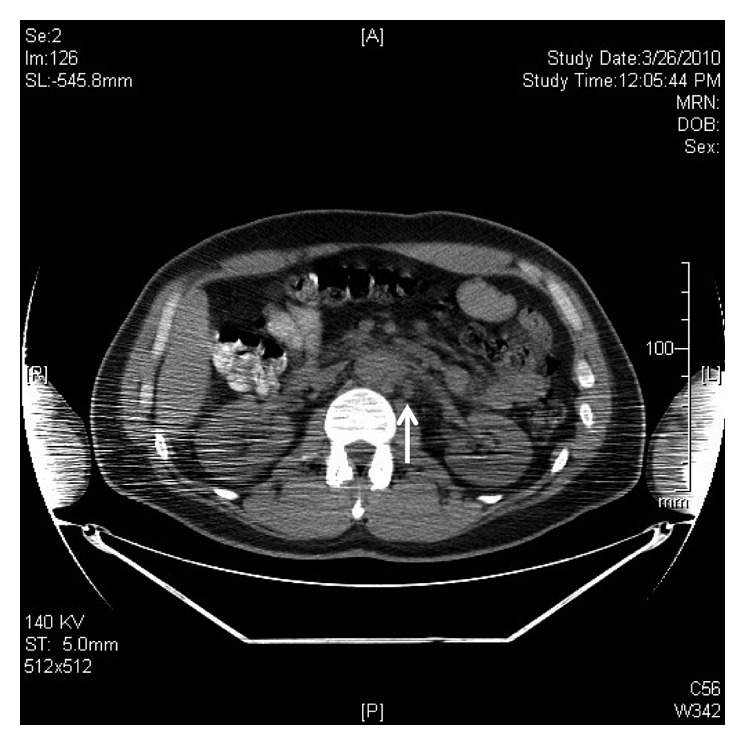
CT image of periaortic lymphadenopathy.
